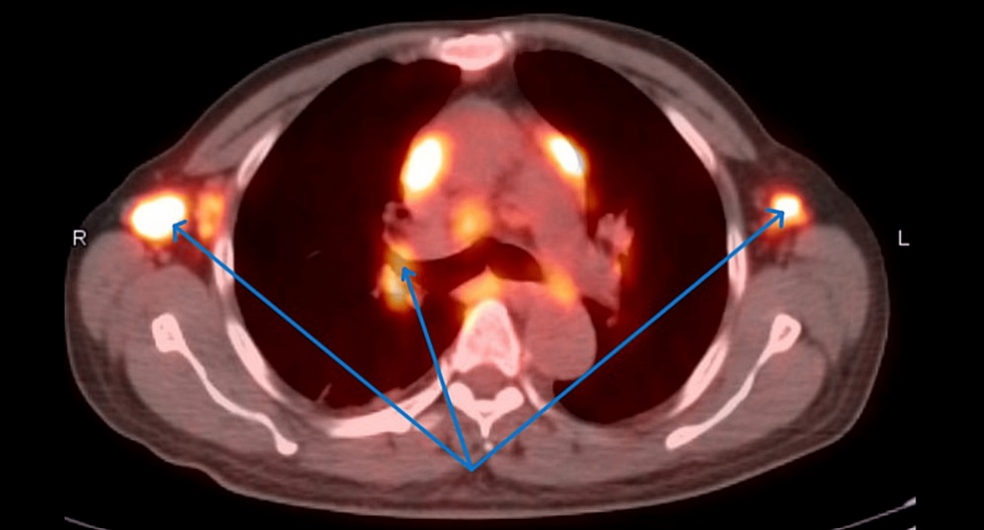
FDG PET/CT showing extensive hypermetabolic disease with bulky mediastinal and axillary lymphadenopathy. FDG PET/CT: F-18-fluorodeoxyglucose positron emission tomography/computed tomography.
